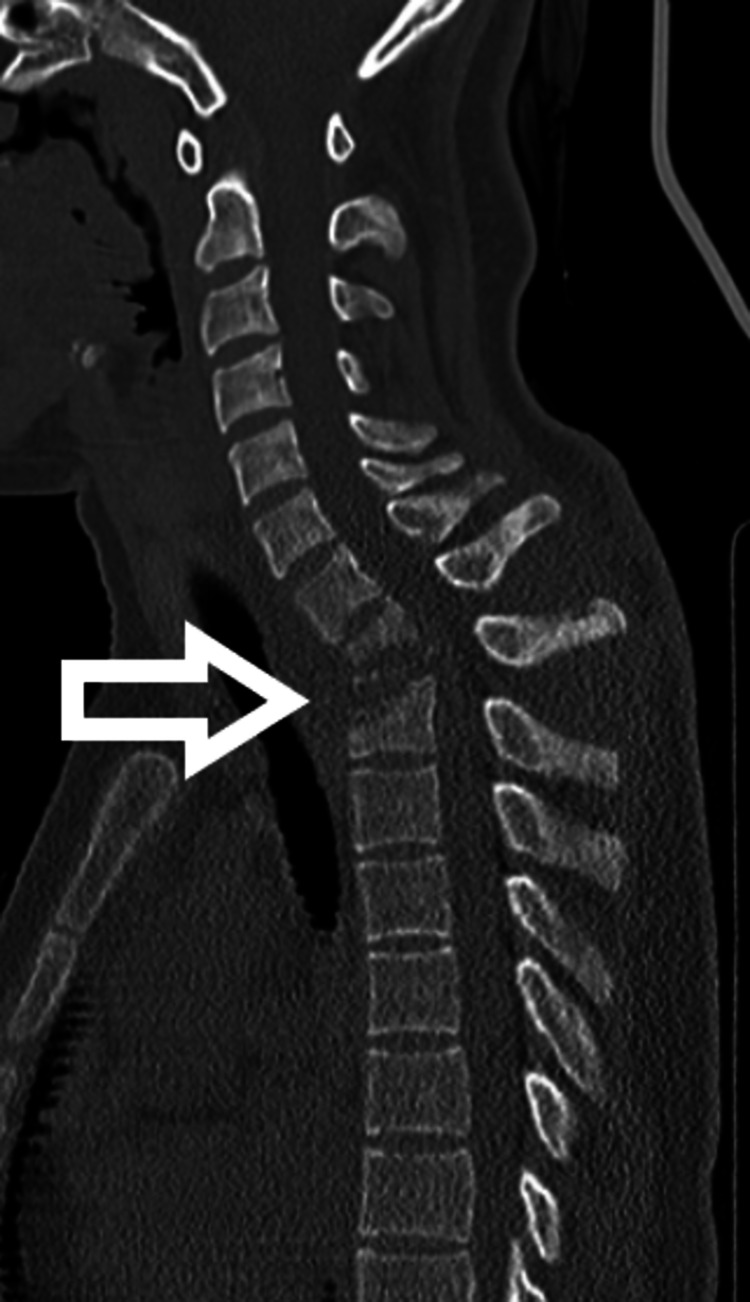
Pre-operative CT Scan showing the involved region
